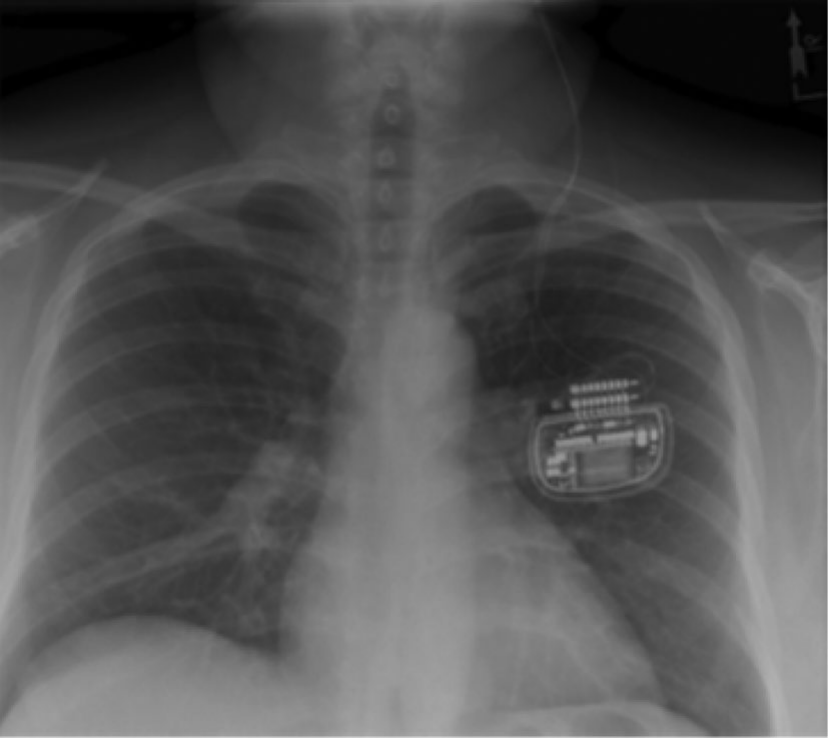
X-ray chest anteroposterior view showing the course of the lead down to the IPG in the generator pocket which is located in the infraclavicular region [10]
